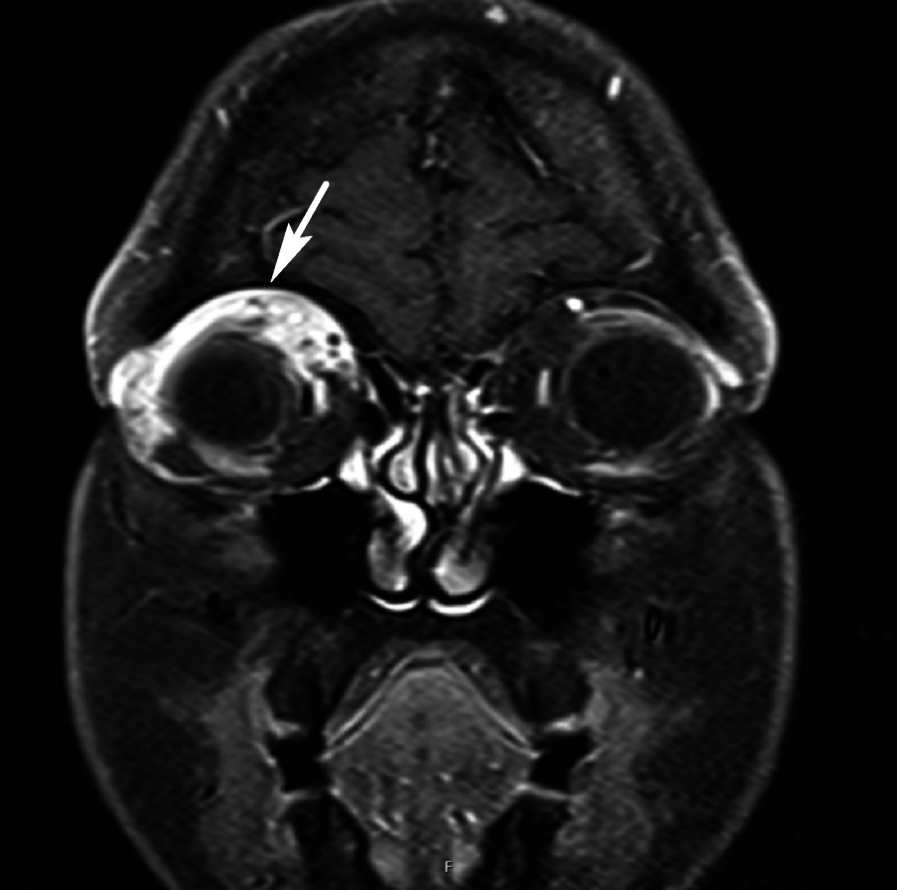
MRI of the head An infiltrative process is noted on MRI of the head in the right orbit (arrow) involving the adjacent muscles and sparing the optic nerve, hypervascular in nature MRI: magnetic resonance imaging
